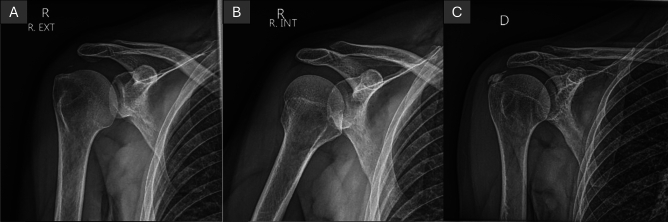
Representative shoulder radiographs showing calcific tendinopathy of the rotator cuff in different projections: (A) external rotation, (B) internal rotation, and (C) anteroposterior views. *CTRC* calcific tendinopathy of the rotator cuff, *ER* external rotation, *IR* internal rotation, *AP* antero-posterior, *XRs* X-rays.
